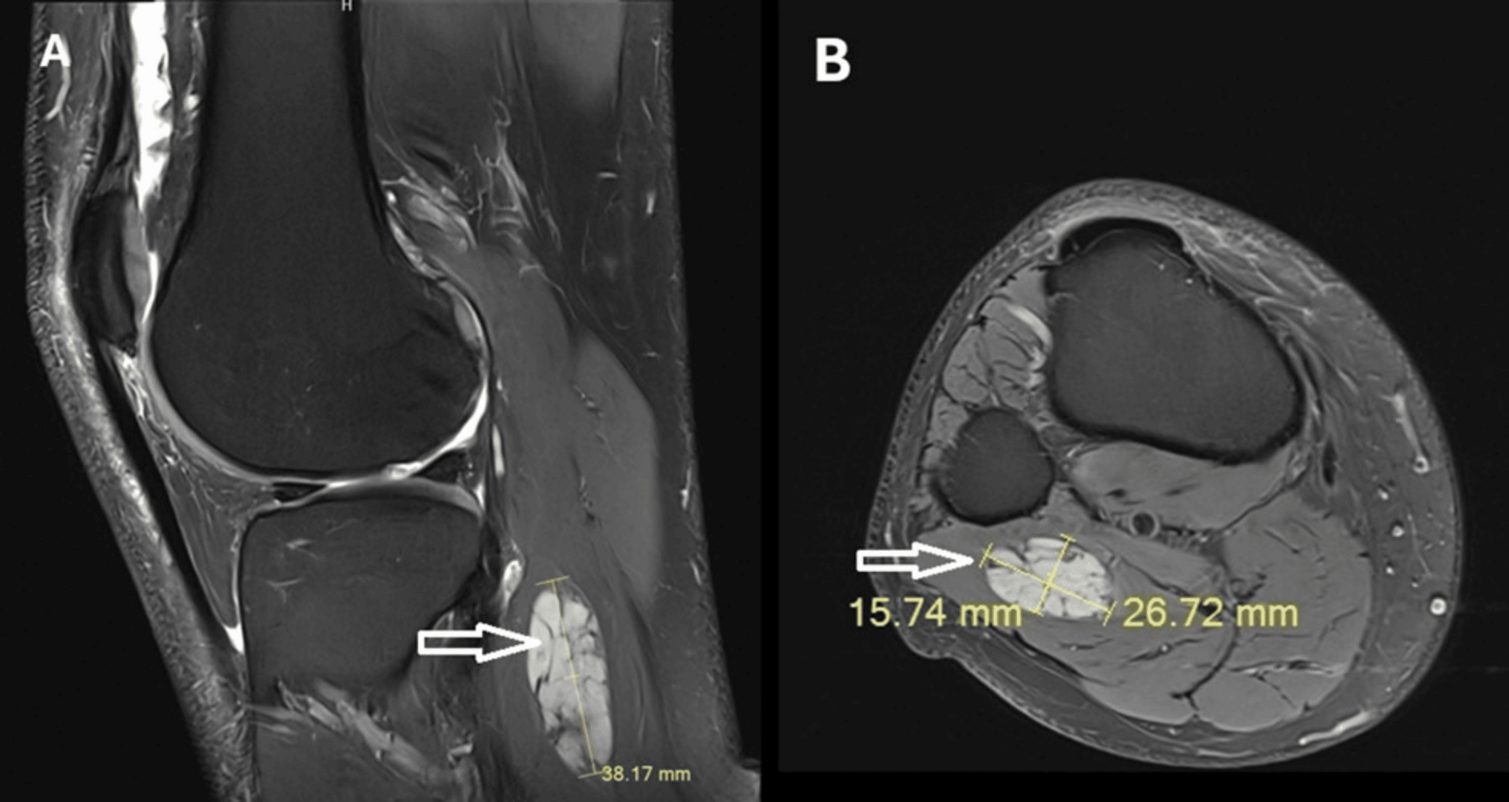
Magnetic resonance imaging (MRI) A: sagittal, B: axial T2-weighted MRI of the right lower leg demonstrating the tumor mass (arrows) in the gastrocnemius muscle measuring up to 38.17 mm.
